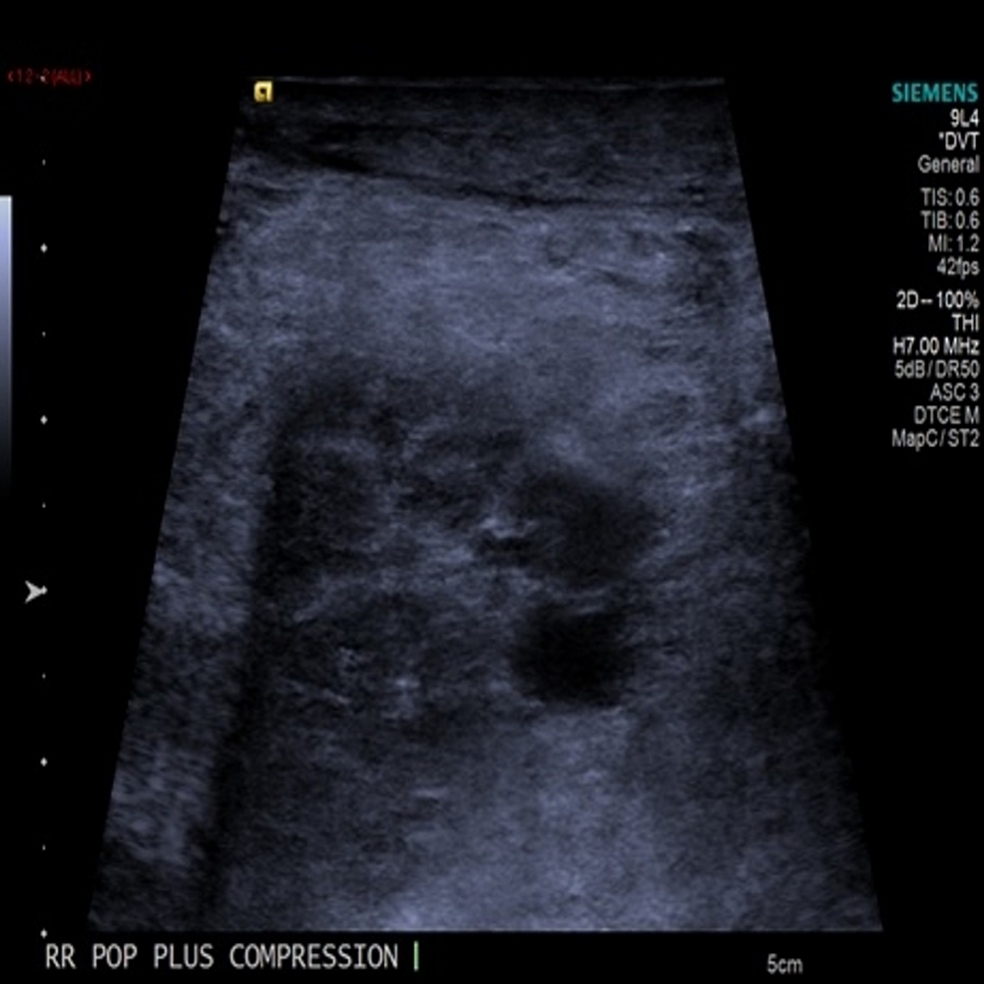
Venous Doppler sonography of the right lower limb; the right popliteal vein is distended with echo poor thrombus
